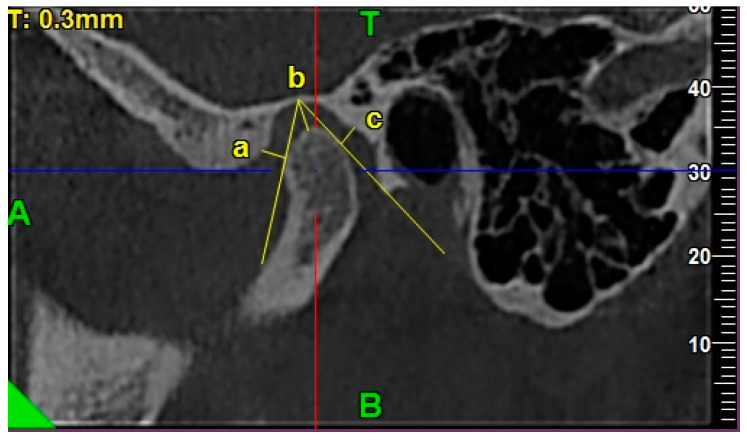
The exemplary lines, points, and angles in the TMJ CBCT scans used for measurements regarding the assessment of anterior, posterior, and superior joint spaces in the sagittal view: /a/ anterior joint space, /b/ superior joint space, and /c/ posterior joint space.
